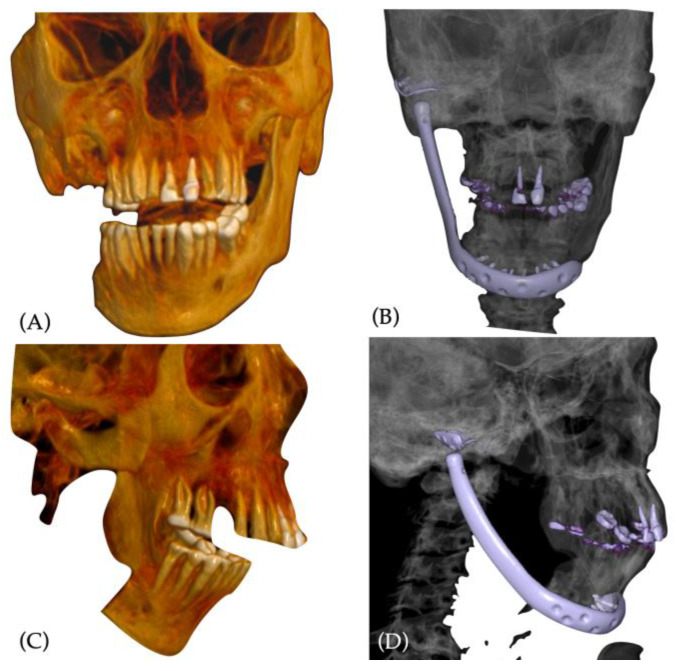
Preoperative and postoperative 3D CT scan comparison: (A,B) coronal view comparison of the preoperative and postoperative CT scan 3D reconstruction, showing the large bone defect filled by the prosthesis positioning and facial harmonization; (C,D) right-side view of the preoperative and postoperative CT scan 3D reconstruction showing prosthesis profile and bettering in occlusion.
